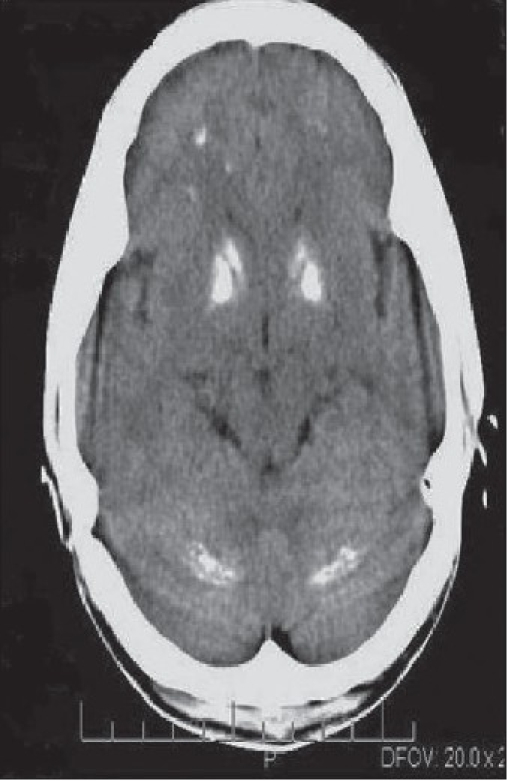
Plain CT head shows symmetrical dense calcification of the anterior lentiform nucleus, anterior limb of the internal capsule, the dentate nucleus of cerebellum, and the gray matter–white matter interface of the frontal lobe on both sides
